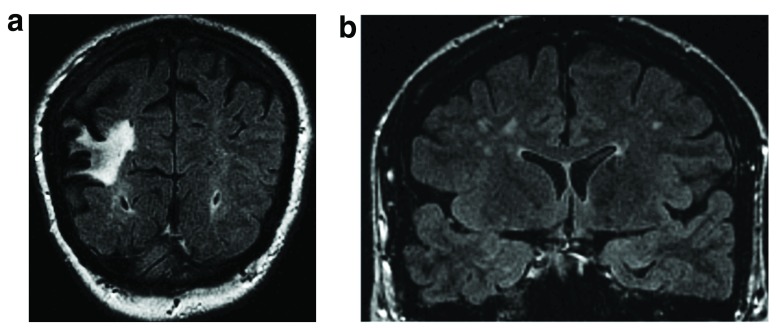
Coronal FLAIR images demonstrate both large and small vessel disease. 1a Large hyperintense cortico-subcortical area consistent with a chronic stroke involving the right middle cerebral artery territory.
